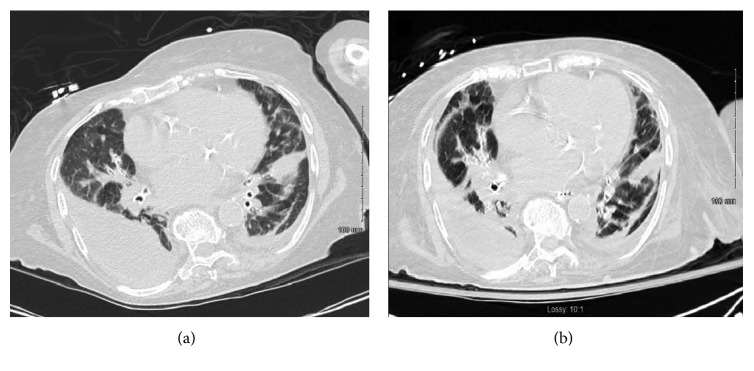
(a) CT chest showing bilateral empyema (right more than left). (b) CT chest showing persistent bilateral empyema after attempted drainage through chest drains.
